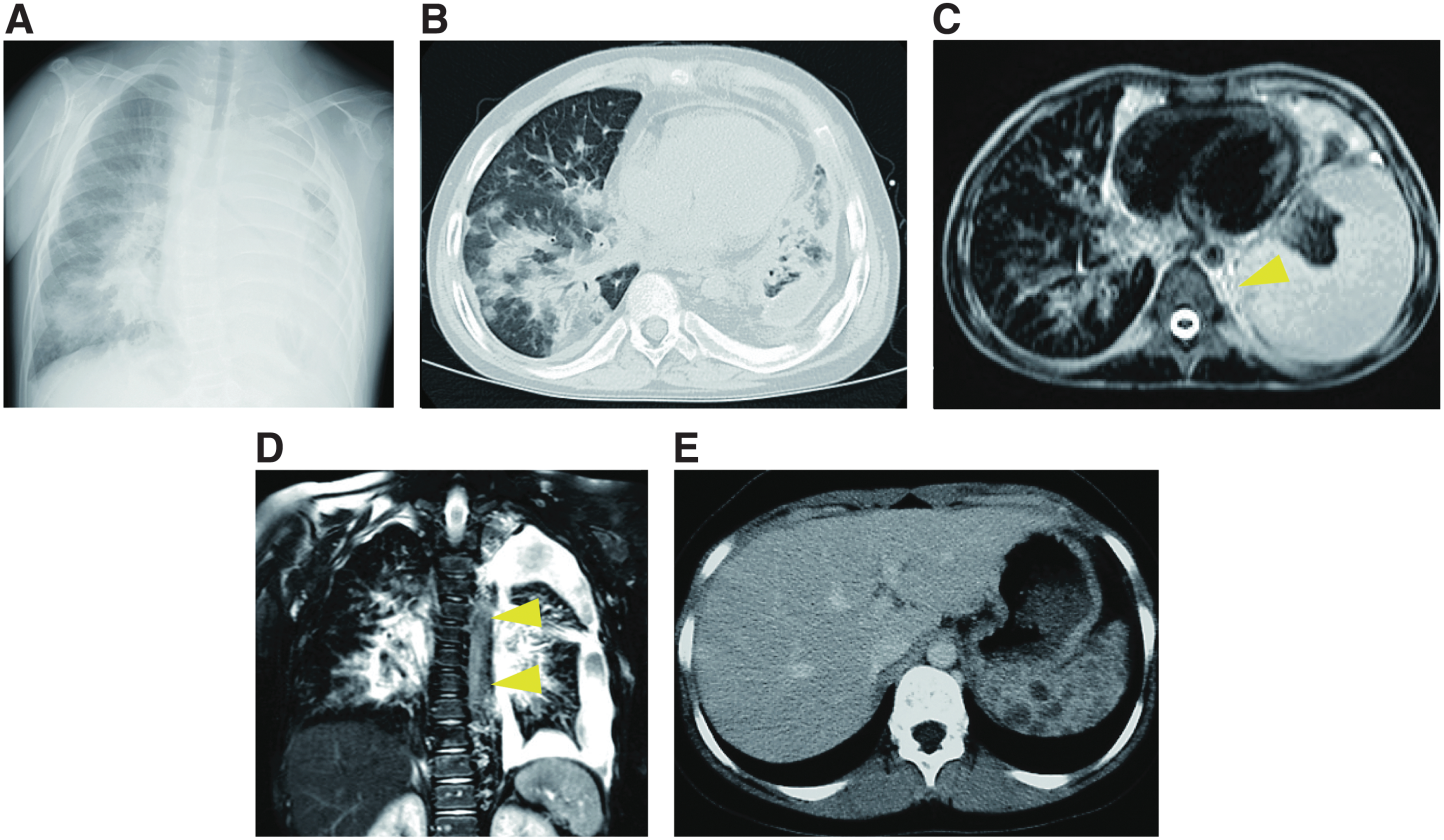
Radiological images of visceral lesions in KLA patients. KLA patients often have mediastinal masses and hemorrhagic pericardial and pleural effusion. In radiological examination, thickening of bronchovascular bundles, retroperitoneal infiltrative soft-tissue thickening, and interlobular septa are common findings. (A–E) A 10-year-old male with KLA. He had a mediastinal mass, hemorrhagic pericardial and pleural effusion, and severe coagulopathy. (A) Plain radiograph of the chest showed left pleural effusion. (B) CT (lung window) showing pleural effusion and bilateral thickening of bronchovascular bundles. (C, D) Thoracic T2-weighted MRI in axial and coronal views shows extensive heterogeneous mass (*yellow arrowheads*) images on the left side extending and involving the bronchopulmonary tree and lung parenchyma. (E) Abdominal CT demonstrating multiple lesions in the spleen.
